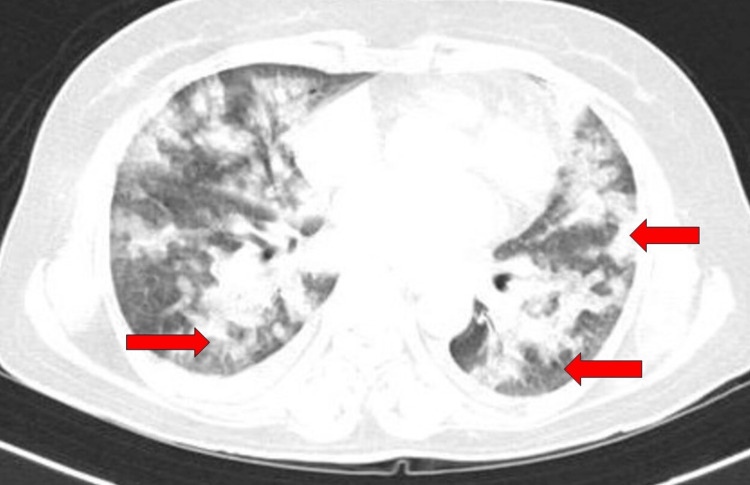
HRCT thorax suggestive of extensive ground glass opacities in the upper and lower lobes of both lungs (red arrow). HRCT: high-resolution computed tomography.
